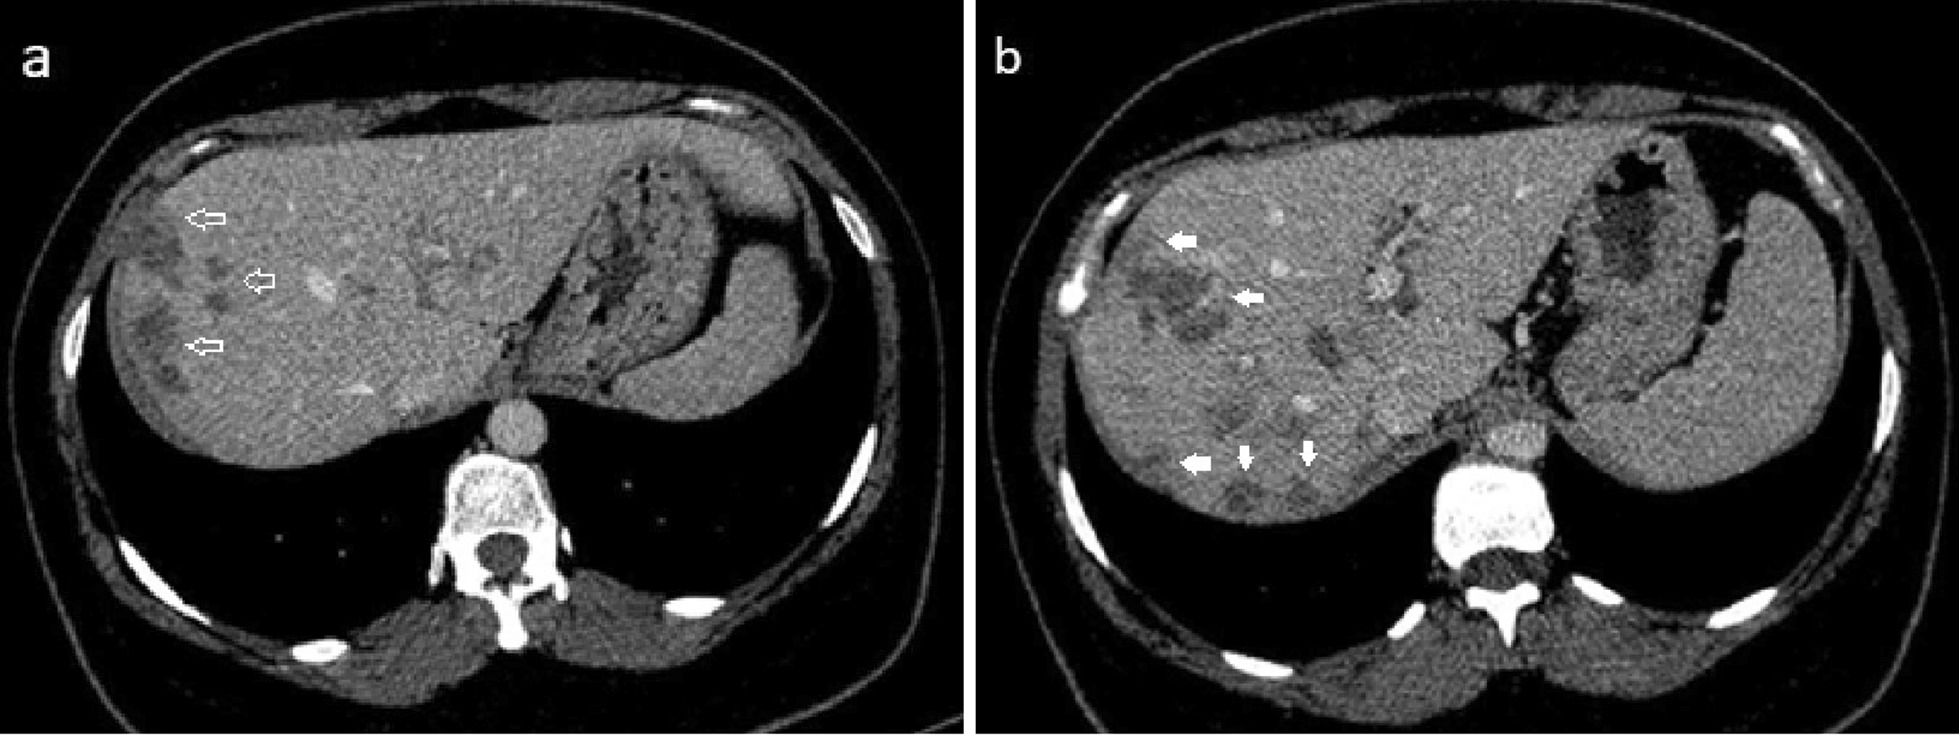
a Axial contrast-enhanced CT-image demonstrating patchy areas of decreased attenuation migrating from the liver capsule into deeper parenchyma and periportal regions showing mild peripheral contrast enhancement (arrows). b Axial contrast-enhanced CT-image demonstrating clusters of hypodense lesions extending from the liver capsule into deeper parenchyma and periportal regions showing mild peripheral contrast enhancement (arrows)
